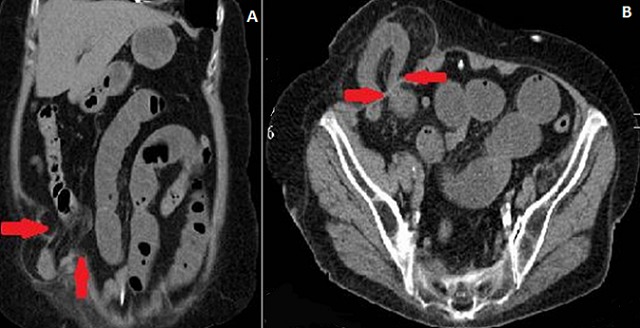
(A) abdominal CT-Scan axial and (B) coronal sections
